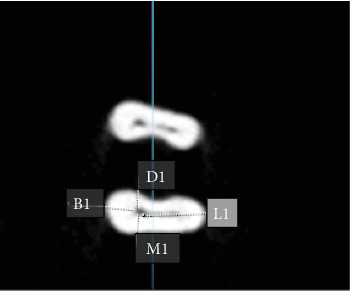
Measurement of dentin thickness on axial cone beam computed tomography (CBCT) sections in the mesial, distal, buccal, and lingual surfaces of the mesiobuccal root.
